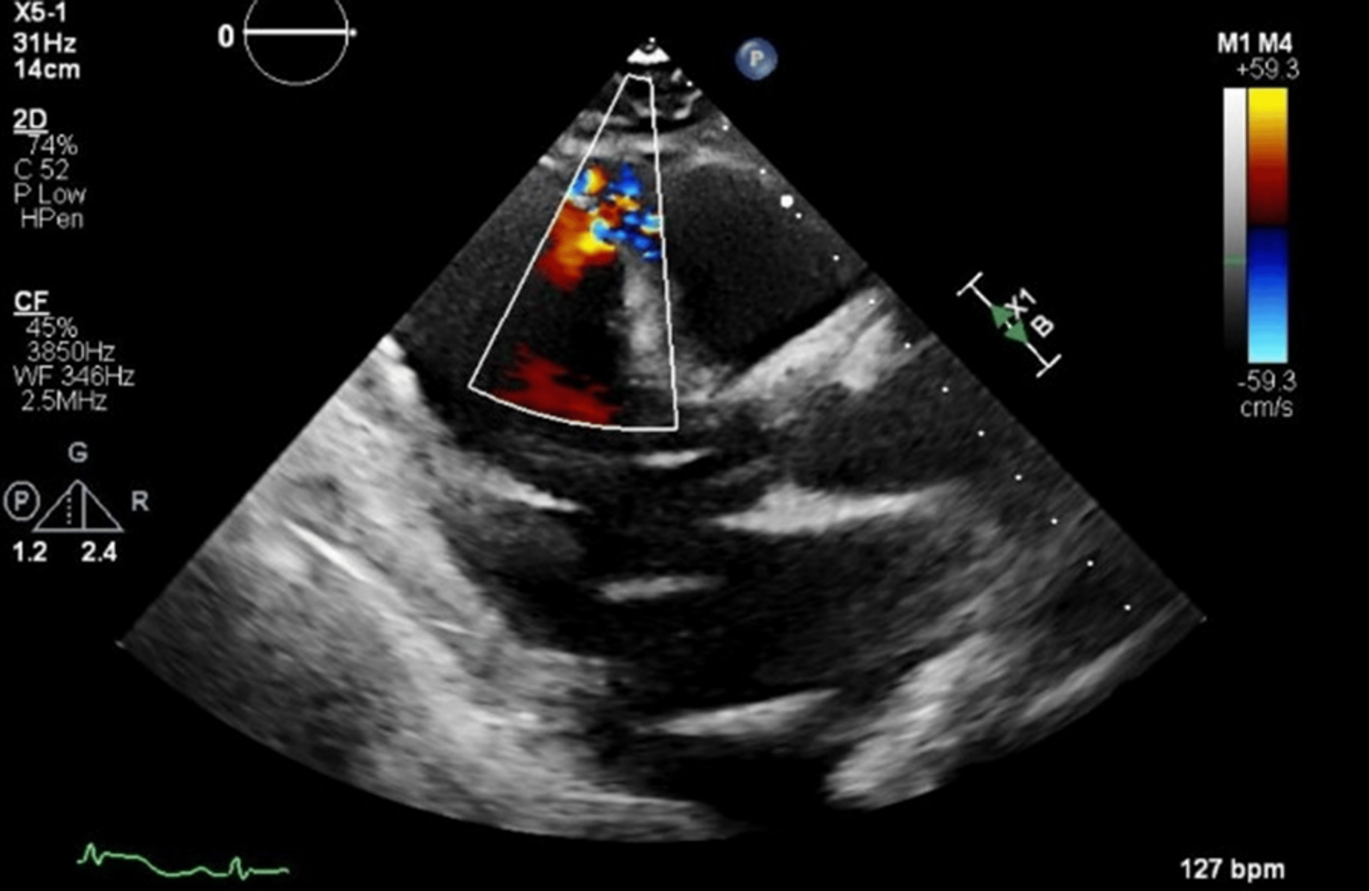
Still image from the patient’s echocardiogram with a large ventricular septal defect (VSD) with left to right shunting towards the apex
